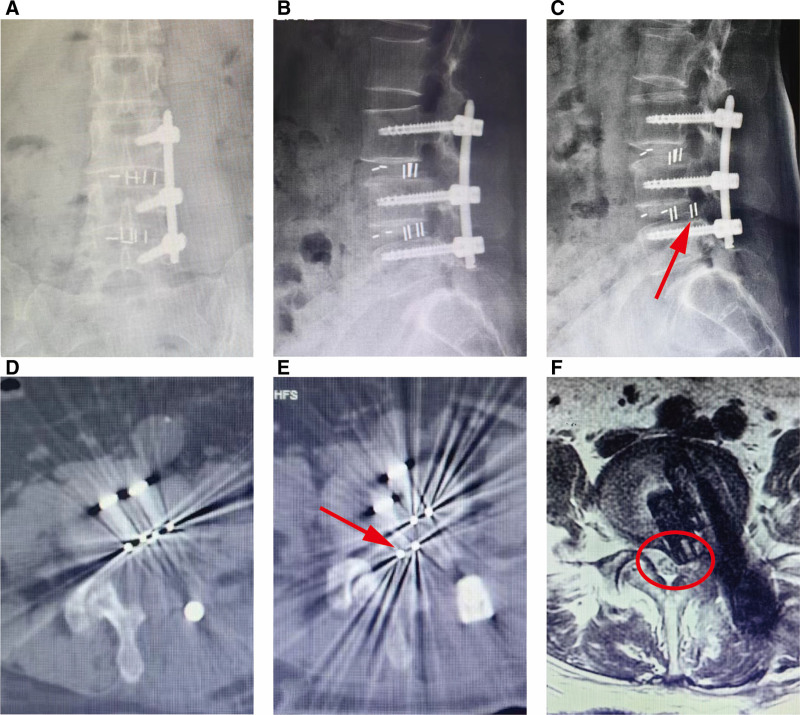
X-ray images at postoperative day 5 (A, B) and 5 weeks (C) after the initial surgery. Cross sectional CT images of the L4-5 segment at postoperative day 5 (D) and 5 weeks (E) following the initial surgery. (F) Transverse MRI scan images of the L4-5 intervertebral space. CT = computed tomography, MRI = magnetic resonance imaging.
